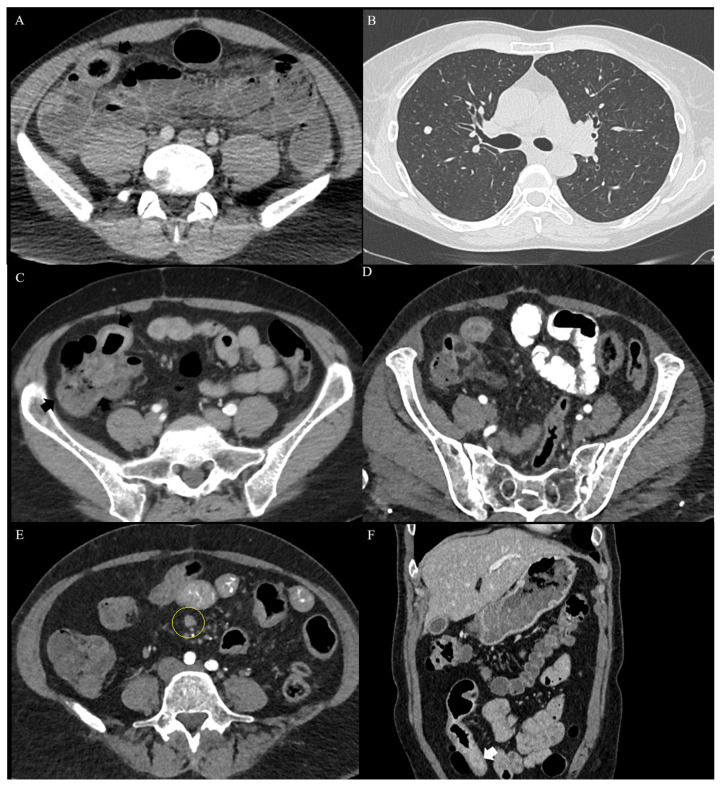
CT scan in patients with ITB (A,B) and CD (C–F). (A) Marked thickening of the ileocecal area (arrow), with dilated bowel loops (intestinal occlusion). Concomitant CT scan has shown pulmonary nodules with fibrosis (B); after bypass surgery, a favorable response to ATT confirmed ITB; (C) inflammatory mass (arrows) located in the ileocecal region (CD). (D) Same case: examination after oral contrast showed small bowel dilation. (E) Same patient: enlarged small abdominal lymph nodes (circle). (F) Coronal reconstruction (same patient) showed ileal stenosis (arrow) with pre-stenotic dilation (CD).
